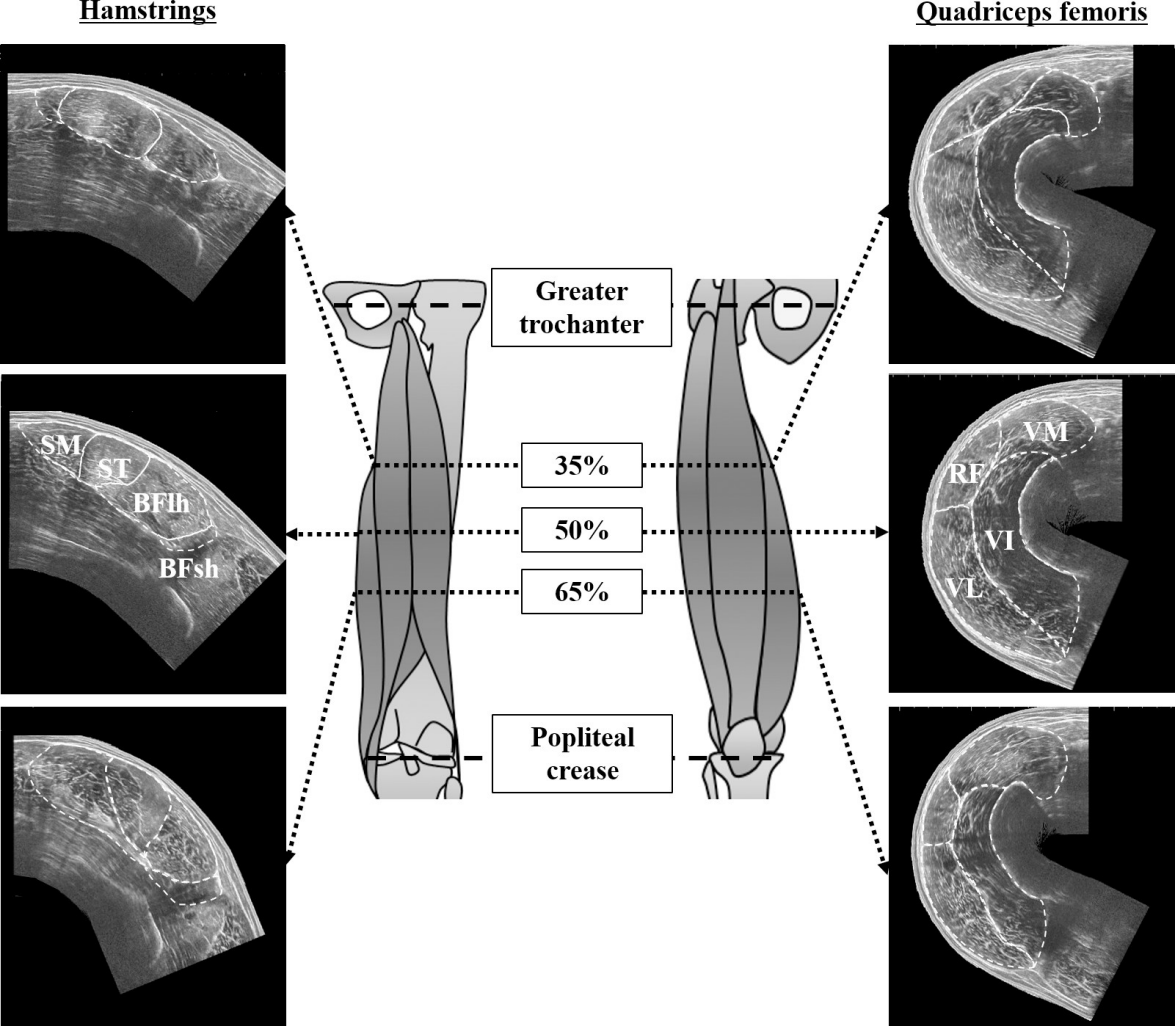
Measurement locations of the anatomical cross-sectional area and ultrasound images in the thigh muscles. The white broken line indicates the outline of the individual muscles of the hamstring and quadriceps femoris. BFlh, biceps femoris long head; BFsh, biceps femoris short head; ST, semitendinosus; SM, semimembranosus; RF, rectus femoris; VL, vastus lateralis; VM, vastus medialis; VI, vastus intermedius.
